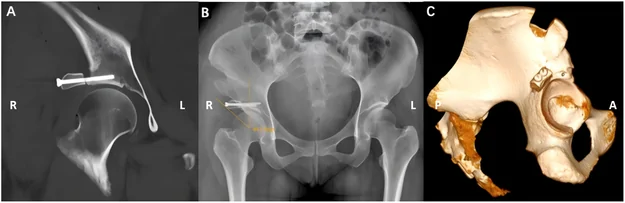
Postoperative imaging of the right hip with the patient in the supine position. (A) Coronal CT scan of the hip joint: The graft bone block matches the curvature of the acetabulum. (B) Radiograph measurement showing a CE angle of 49.1° after fixation. (C) 3D reconstruction of the hip joint CT. (3D, 3‐dimensional; CE, center‐edge; CT, computed tomography.)
